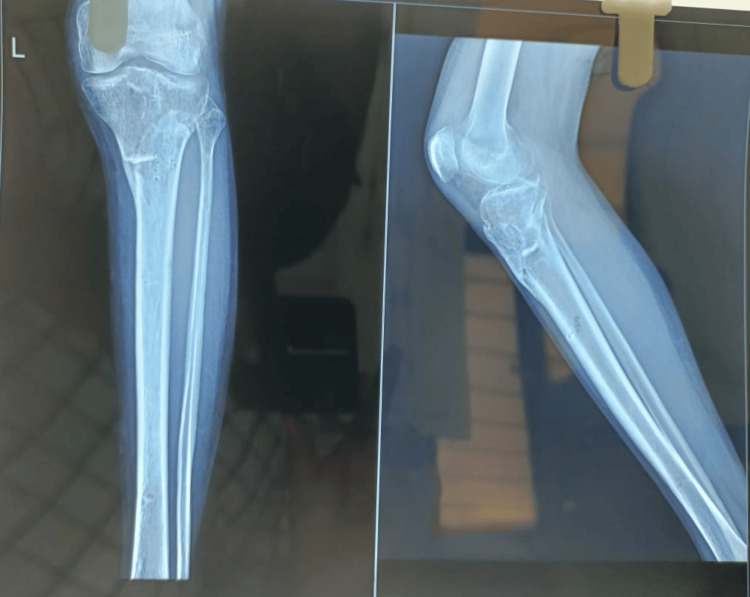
X-ray after removal of Ilizarov construct after achieving radiological union at the fracture site
